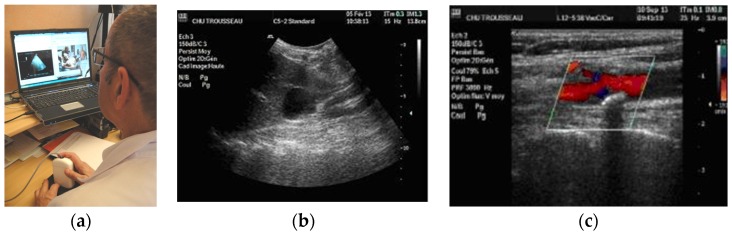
(a) RA expert center computer screen with video of the patient (left) and echographe screen (right); (b) Renal cyst image obtained using RA; (c) Carotid bifurcation with atheromatous plaque in color Doppler using RG.
